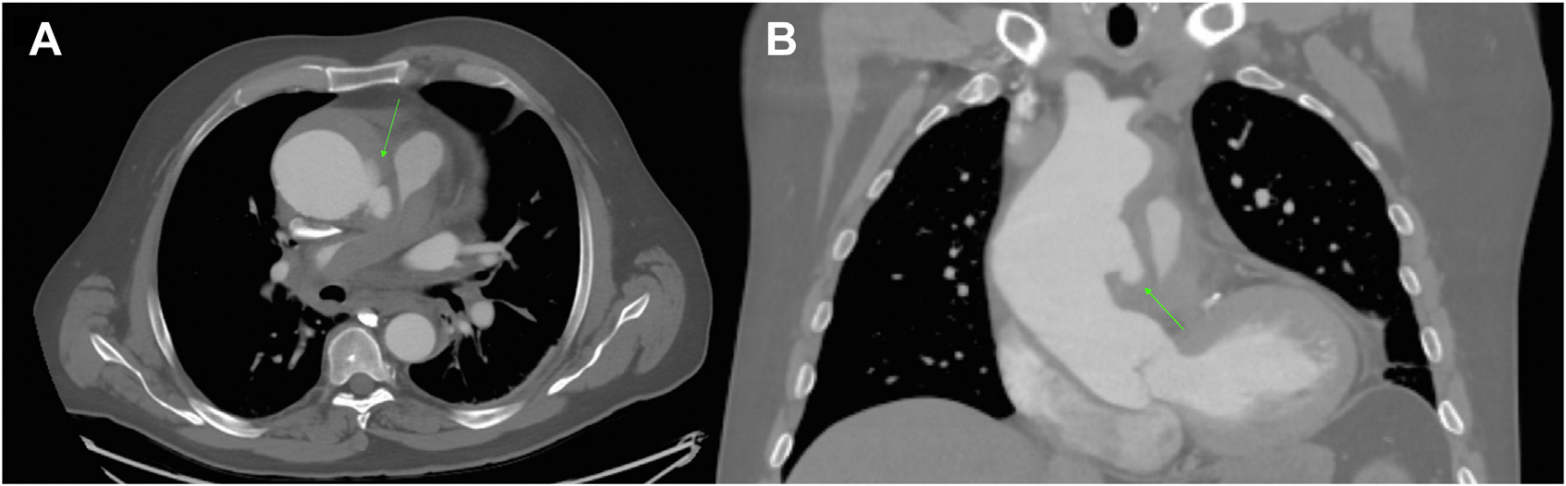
(A) Axial and coronal (B) contrast-enhanced computed tomography image demonstrating a focal aortic tear and rupture along the left lateral aspect of the mid-ascending aorta (green arrows) into the shared adventitial sheath of the great vessels.
